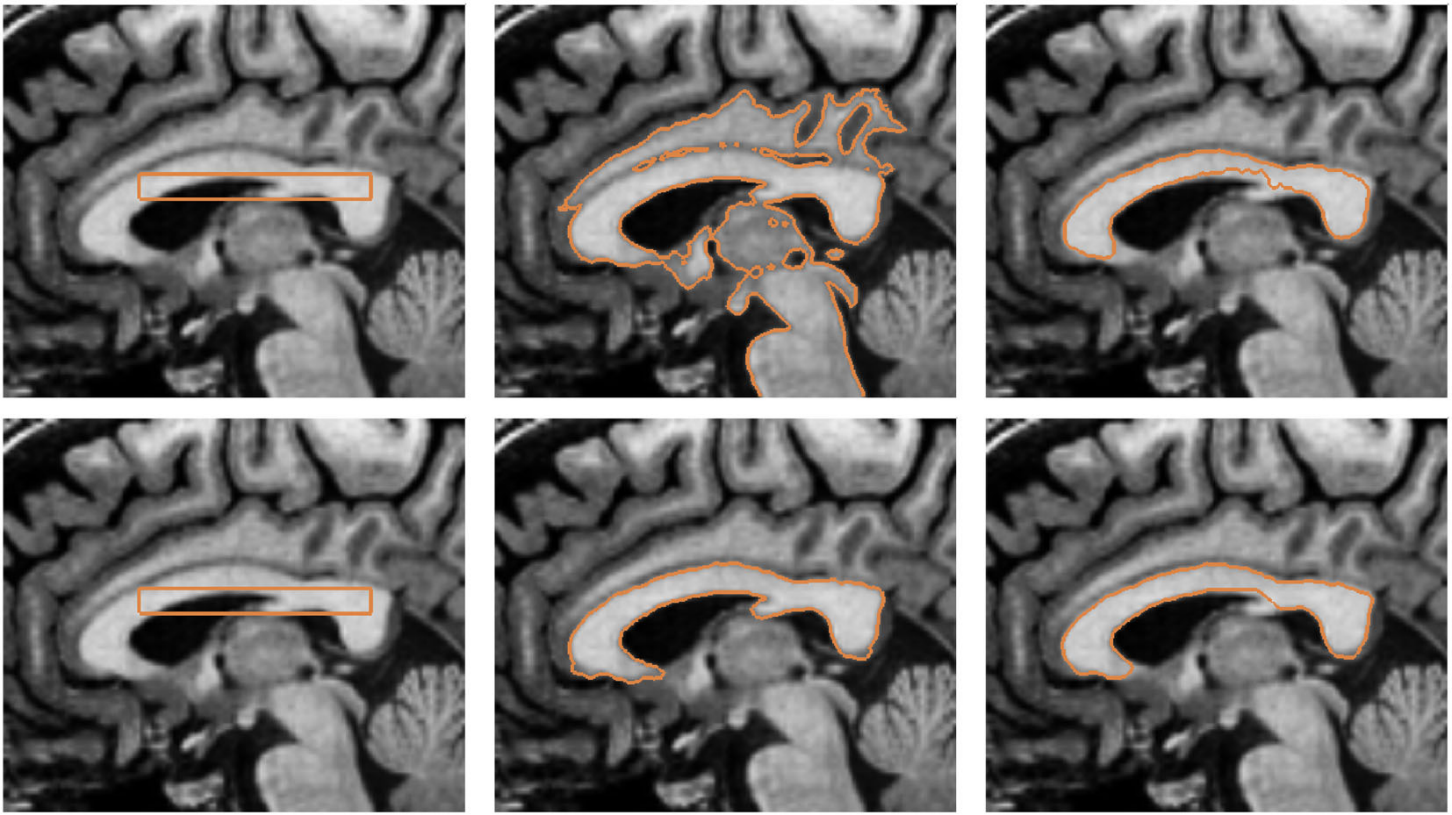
Segmentation of corpus callosum from magnetic resonance image: (top row, from left to right) initial contour, Chan-Vese model, Chan-Vese model with shape prior, (bottom row, from left to right) initial contour, gradient vector interaction-based energy, gradient vector interaction, and shape-based energy.
